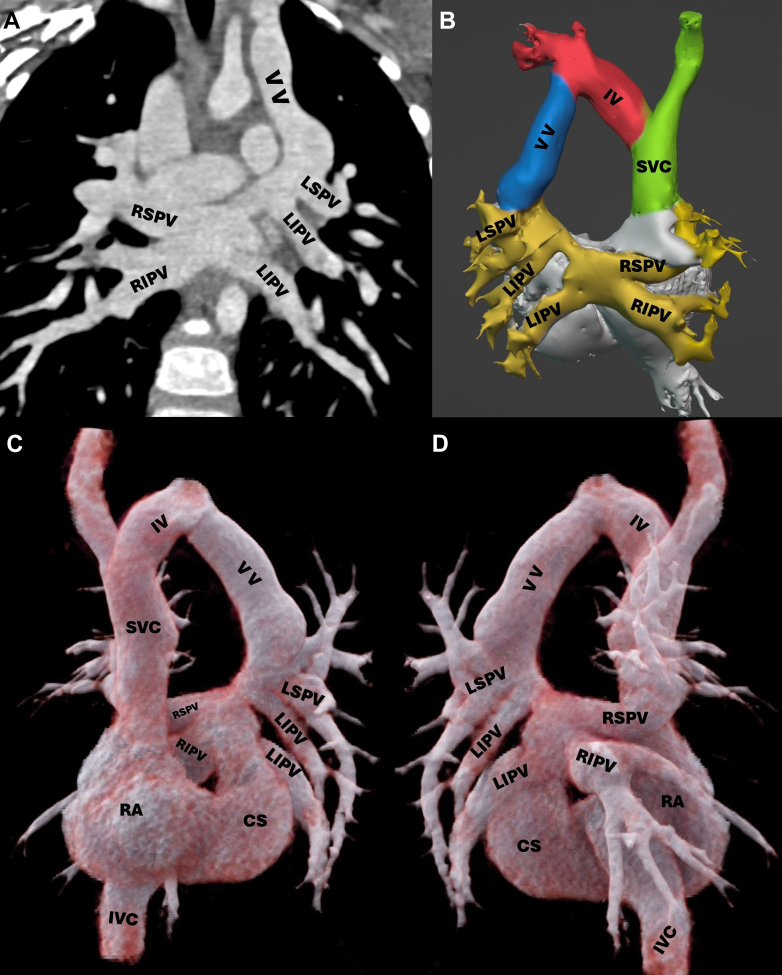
Patient 2 With Dual Drainage Total Anomalous Pulmonary Venous Connection CCTA showing drainage of the right and left pulmonary veins to a common collector, which flows to the coronary sinus and SVC via a VV connected to the innominate vein. (A) Coronal with MIP view. (B) VRT 3D reconstruction imaging in posterior view. (C, D) Cinematic VRT 3D reconstruction imaging in anterior and posterior views, respectively. 3D = 3-dimensional; CCTA = cardiac computed tomography angiography; CS = coronary sinus; IV = innominate vein; IVC = inferior vena cava; LIPV = left inferior pulmonary vein; LSPV = left superior pulmonary vein; MIP = maximum intensity projection; RA = right atrium; RIPV = right inferior pulmonary vein; SVC = superior vena cava; VRT = volume rendering technique; VV = vertical vein.
